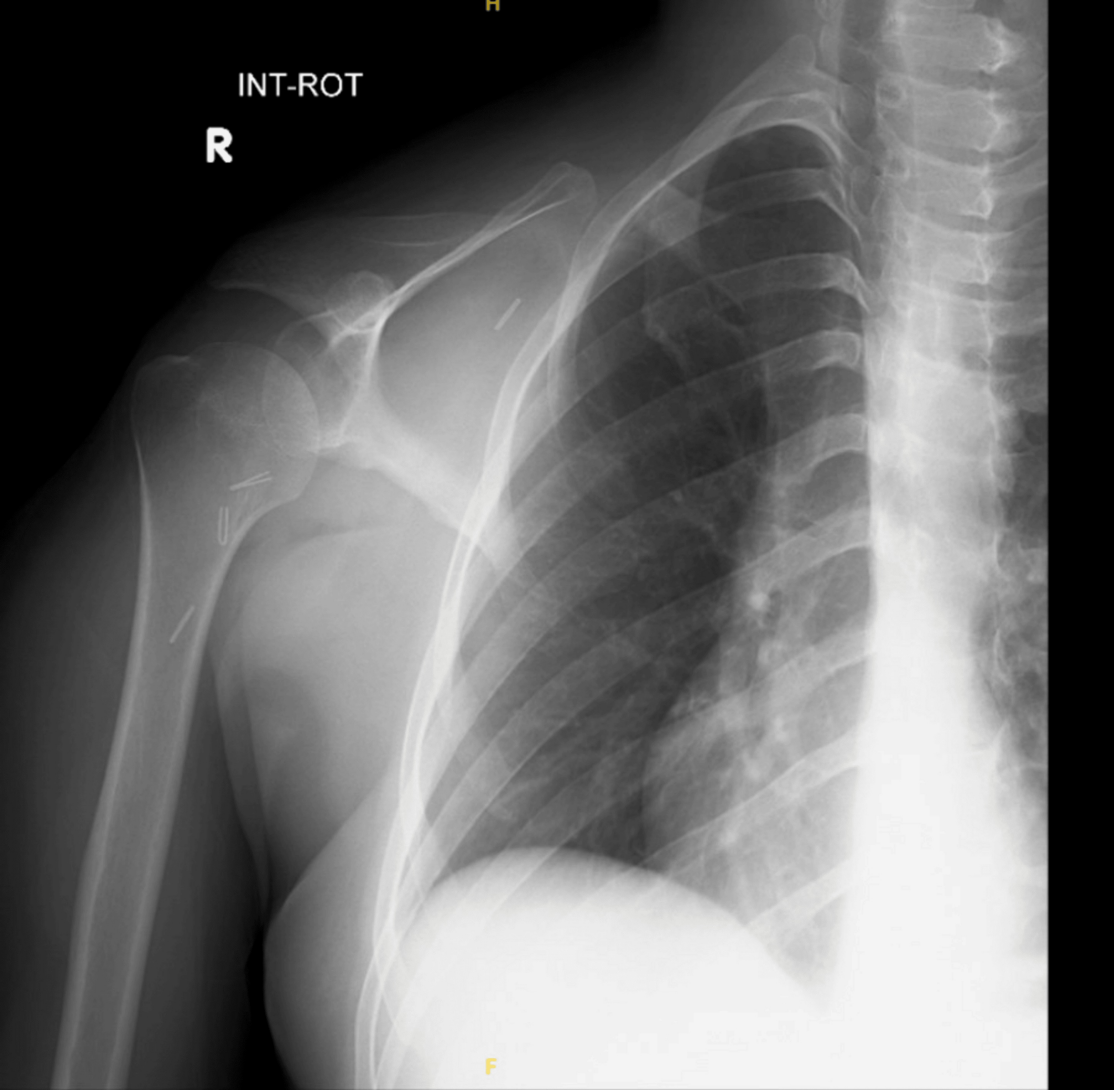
Postoperative X-ray of the patient's right shoulder showing the successful resection.
